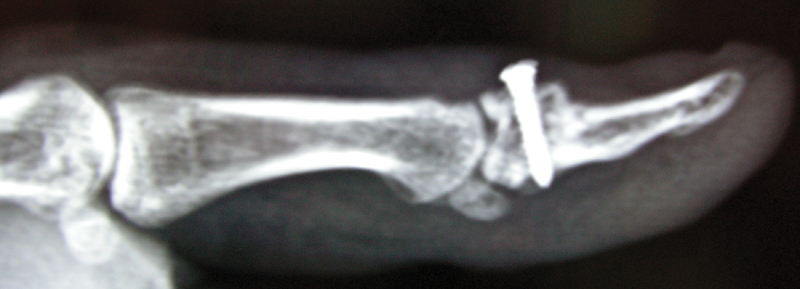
Six months postoperative lateral radiograph.
